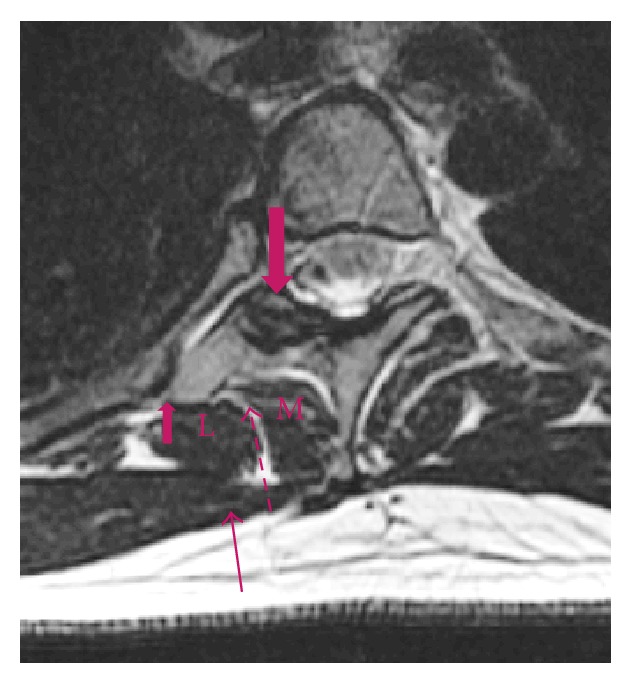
MRI axial image of the thoracic spine showing the facet joint (large block arrow), which is less prominent than the transverse process (small block arrow). The plane of dissection (dashed arrow) is between multifidus medially (M) and longissimus laterally (L). Also notice the fibers running transversely of the trapezius, which lies superficially to the thoracodorsal fascia and has to be incised to expose the surgical plane.
